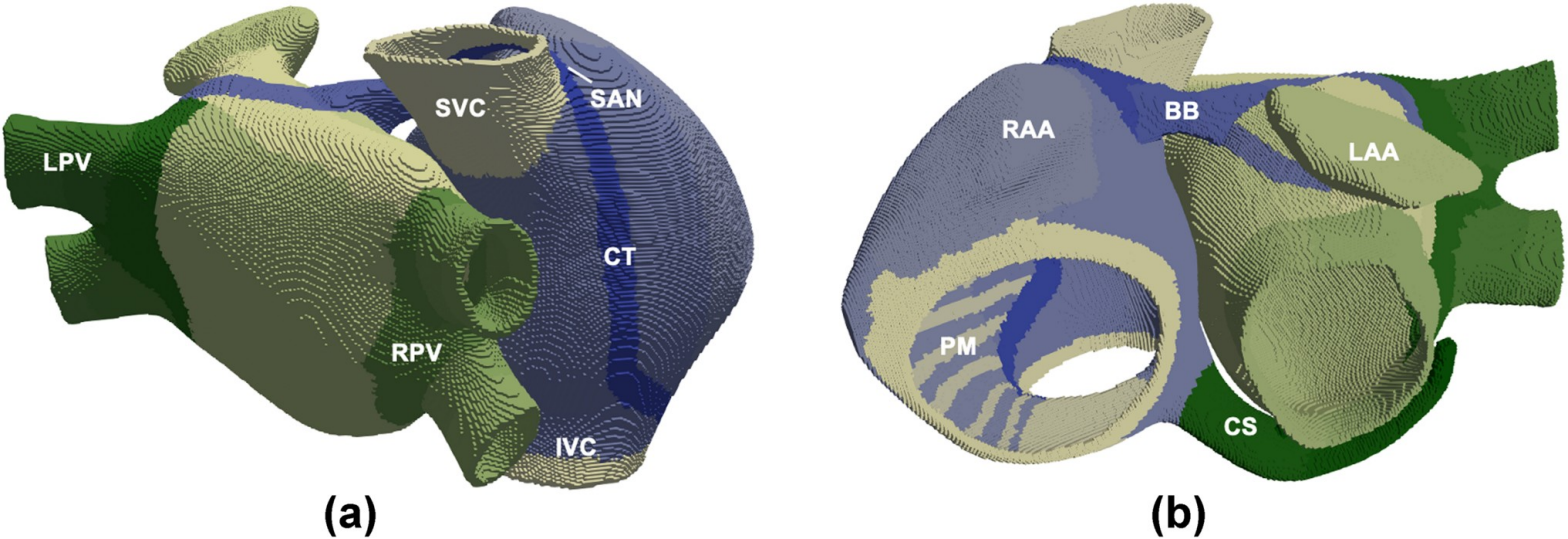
The 3D model of human atria. (a) Frontal and (b) dorsal views. Colored areas show regions with different fiber orientation, conductivity and/or electrophysiological heterogeneity. LAA and RAA: Left and right appendages, PM: Pectinate muscles, BB: Bachmann’s bundle, CS: Coronary sinus, LPV and RPV: Left and right pulmonary veins, SCV and ICV: Superior and inferior caval veins, CT: Crista terminalis, and SAN: Sinoatrial node.
